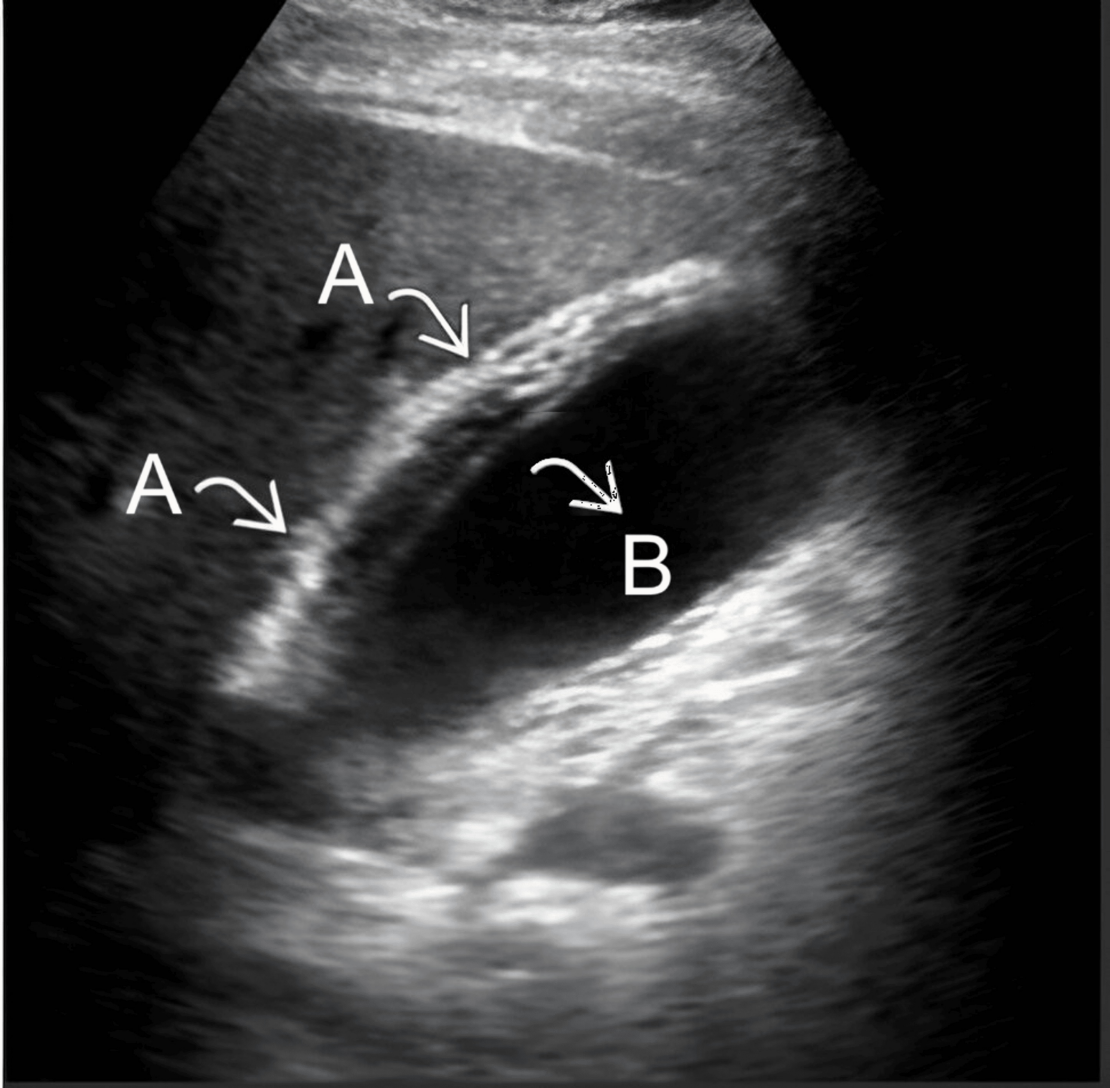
Ultrasound of the liver and biliary tract A) The gallbladder was found with edema, thickening, and stratification of the wall. B) Otherwise, gallbladder distention with absence of gallstones was observed.
